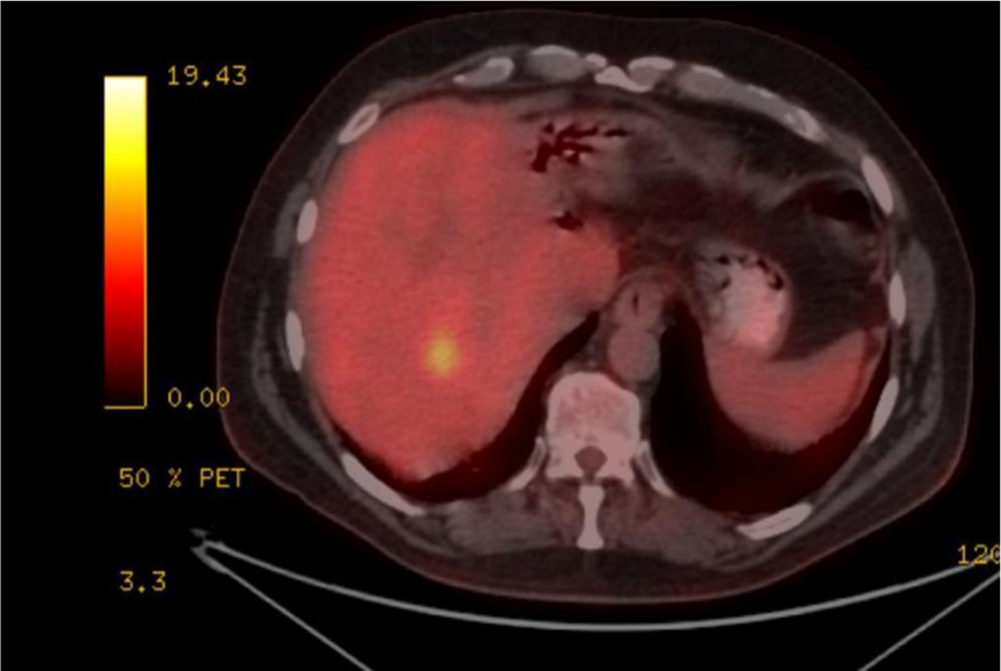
Positron emission tomography/computed tomography indicated FDG-uptake that metastatic-like lesions in the liver.
